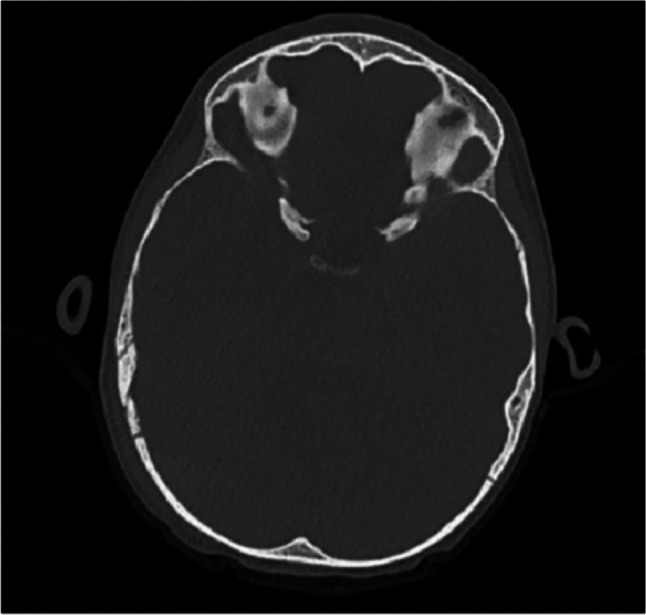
CT bone window of right occipital bone fracture extending to lambdoid and occipitomastoid sutures. The fracture underlies the right subgaleal haematoma, is superiorly undisplaced and inferiorly mildly disrupts the minimally widened lambdoid suture on the right.
